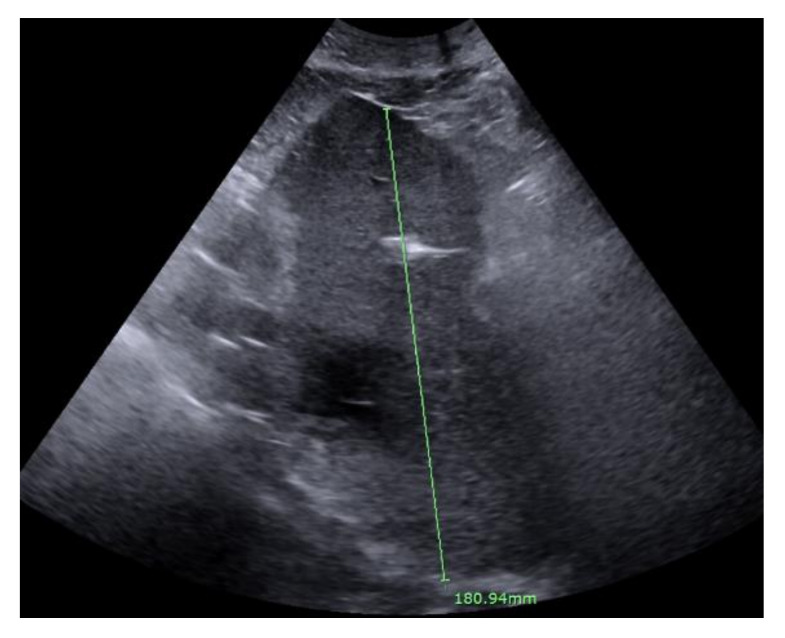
Abdominal ultrasound showing mass-like lesion in the left hepatic lobe.
